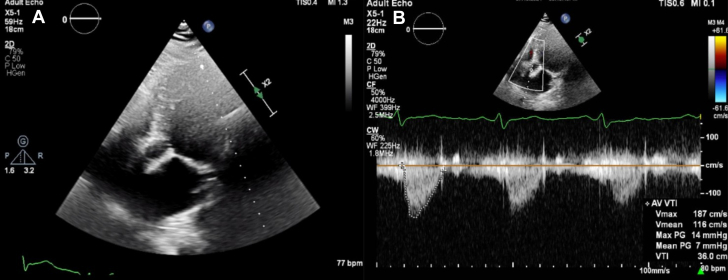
Transthoracic Echocardiography of Redo Transcatheter Aortic Valve Replacement (A) A 2-dimensional (2D) apical 5-chamber view demonstrates the left ventricular outflow tract and the aortic valve (AV), with the previous transcatheter heart valves appearing as echogenic structures. (B) Doppler imaging across the aortic valve confirms proper function of the previous transcatheter heart valves, with no evidence of stenosis or regurgitation. The recorded peak velocity is 187 cm/s, the mean pressure gradient (PG) is 7 mm Hg, and the velocity-time integral (VTI) is 36.0 cm.
